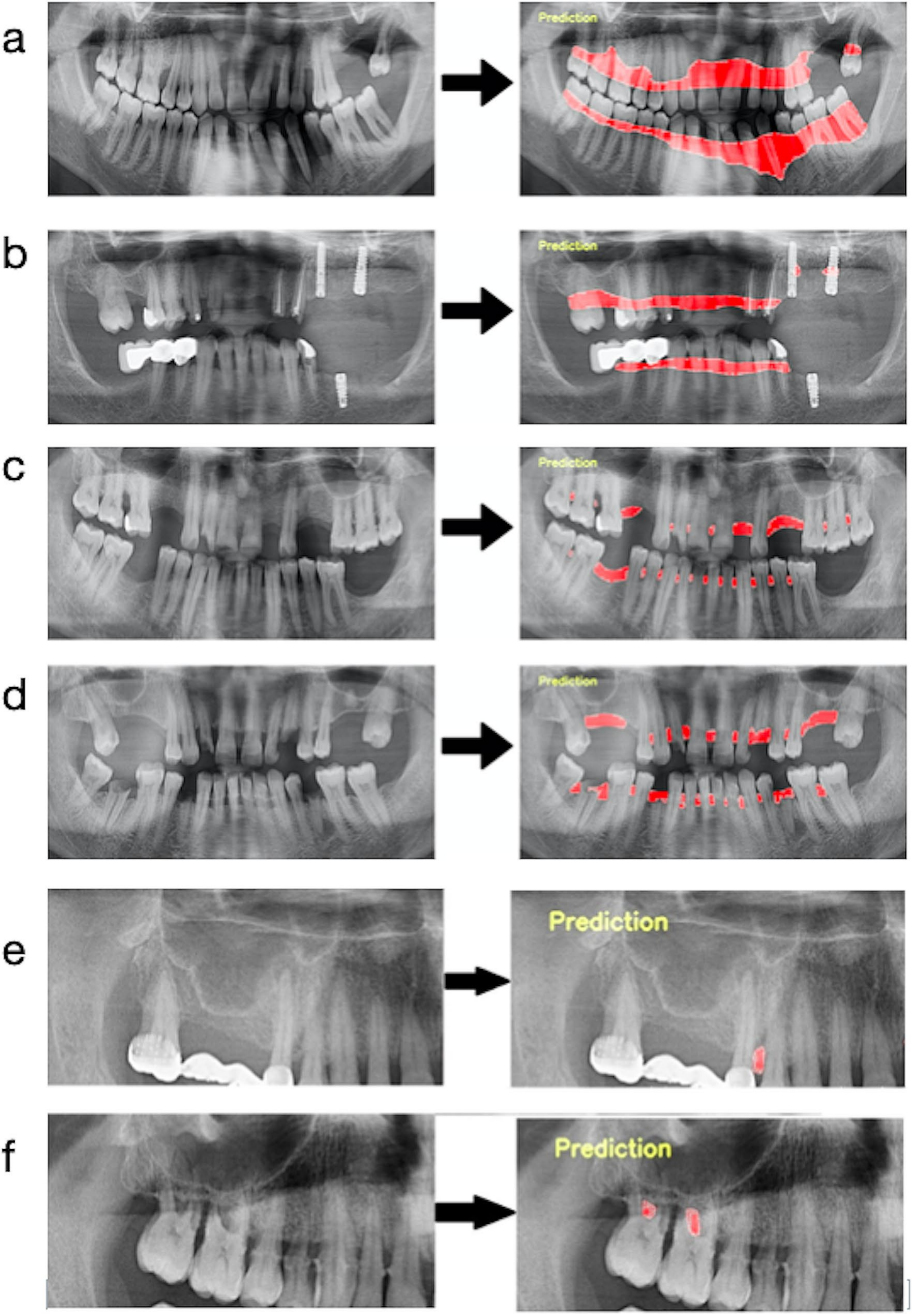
Input and output data of some radiographs of patients. a-b. Total alveolar bone loss; c-d. Horizontal bone loss; e. Vertical bone loss, f: Furcation defect
